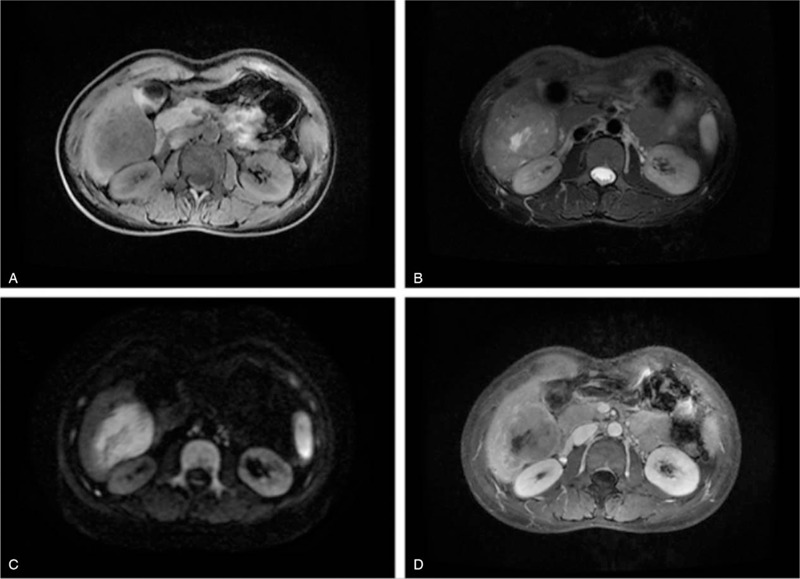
Magnetic resonance imaging (MRI) findings. A, On MRI, the mass in the right liver appeared hypointense on T1-weighted images. B, The mass appeared inhomogeneous hyperintense on T2-weighted images. C, The mass appeared inhomogeneous hyperintense on DWI-weighted images. D, On contrast-enhanced MRI, the mass was slightly enhanced.
